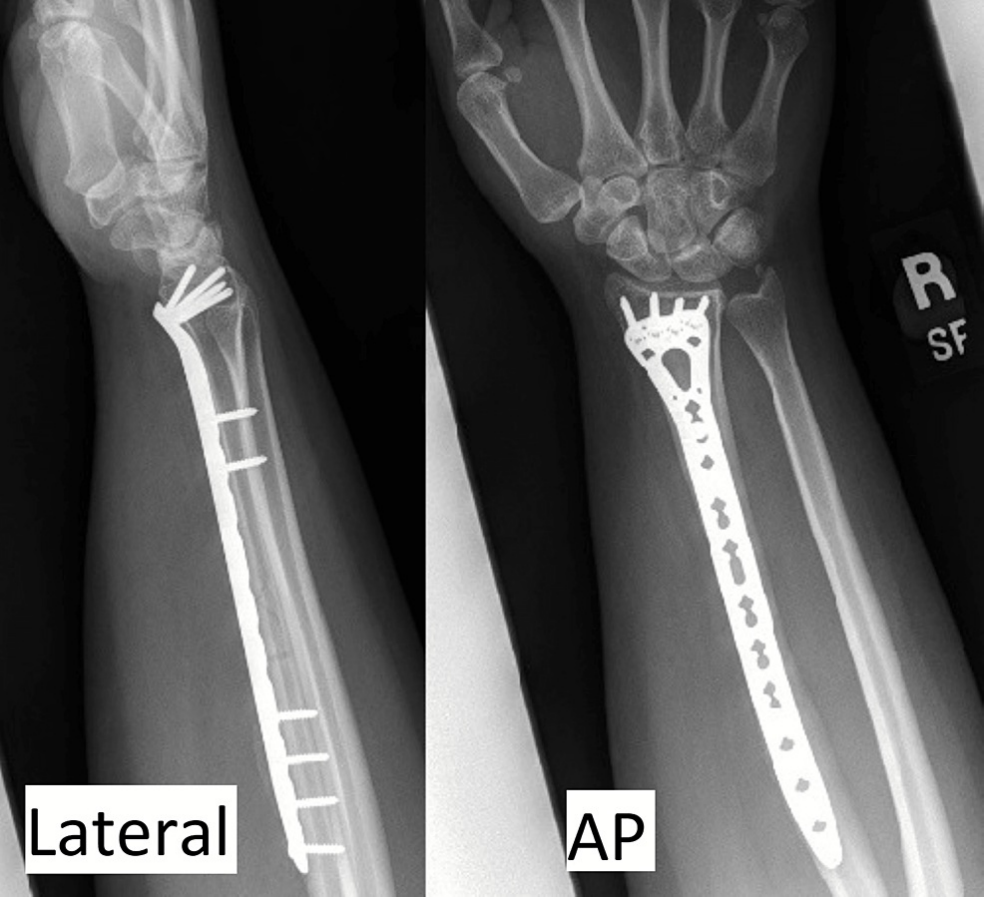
Anteroposterior (AP) and lateral view X-rays of the right forearm demonstrating full healing of radial shaft fracture six months postoperatively
